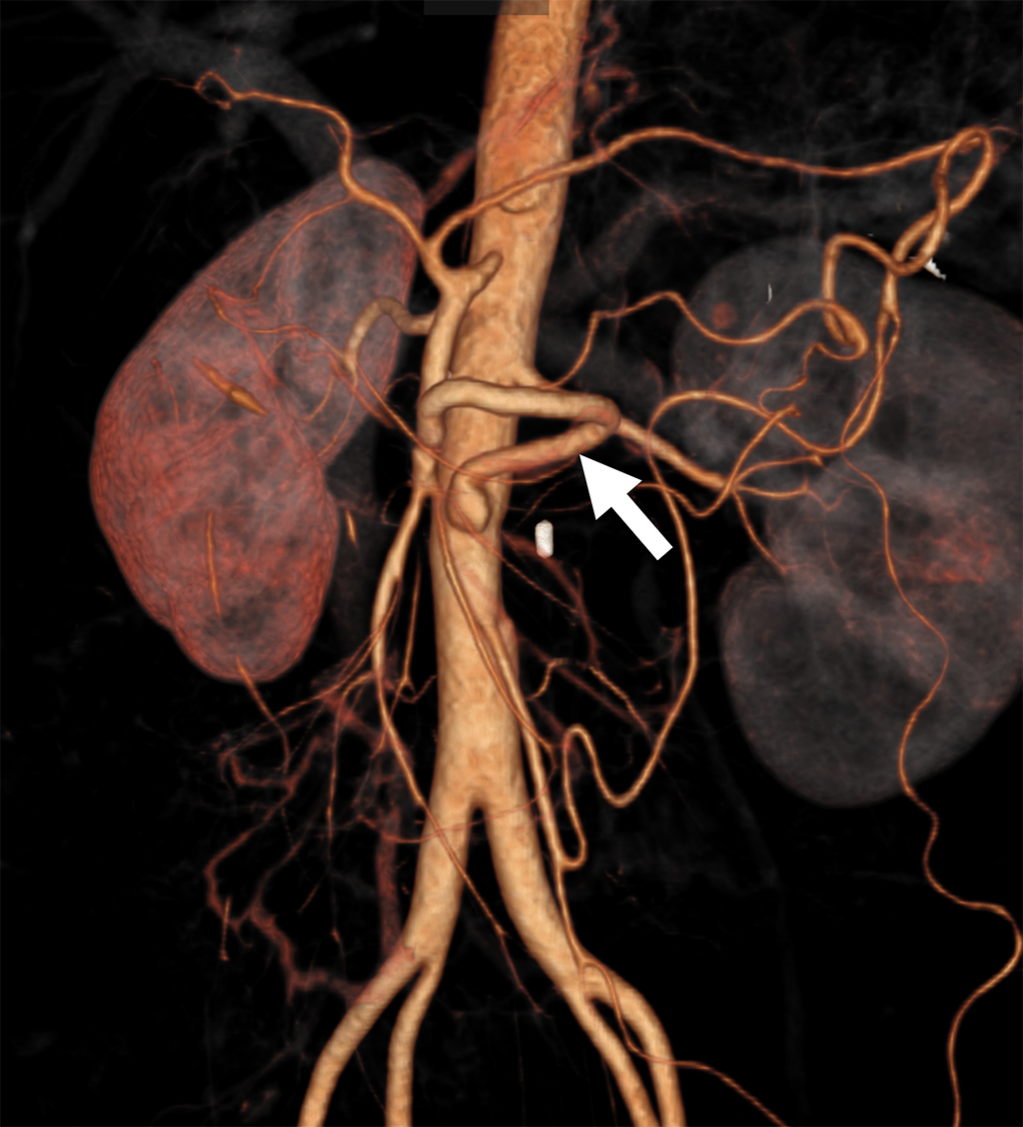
Postoperative computed tomography showing an aorta to superior mesenteric artery bypass (arrow).
